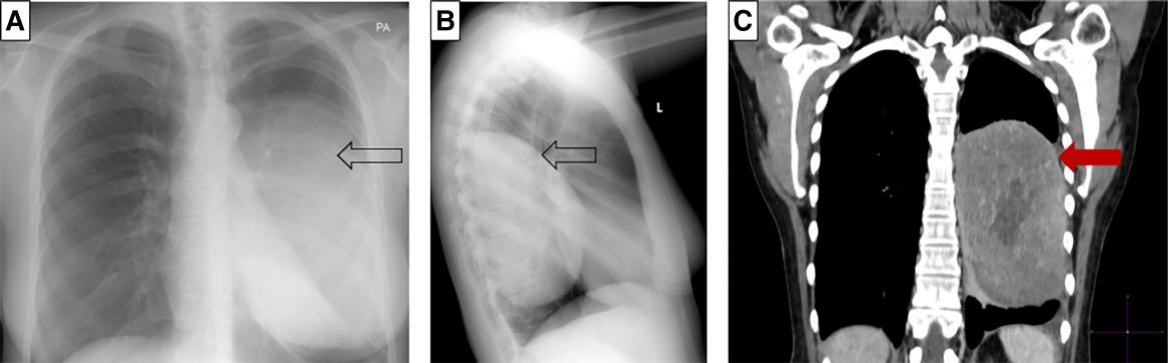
Localization images. A and B, Chest radiograph showing a left pleural–based mass (indicated by arrow): A, posteroanterior; B, lateral. C, Computed tomography of the chest showed a well-delineated, left pleural–based mass measuring 9.7 × 11.8 × 11.8 cm (red arrow).
